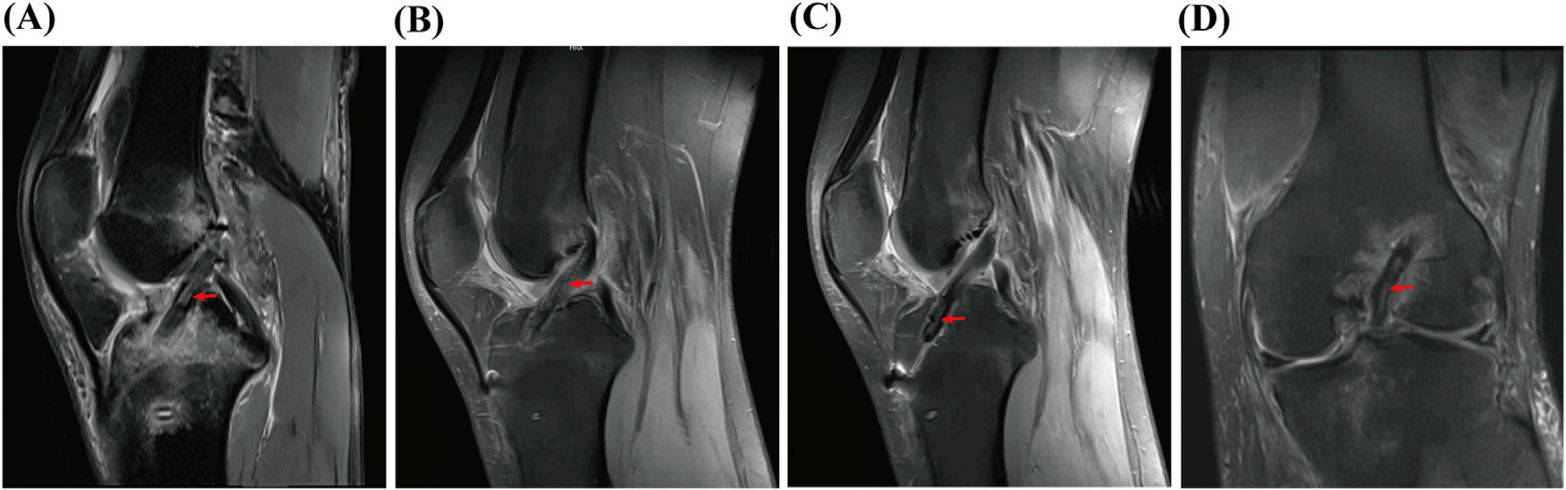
MRI results. (A-C) are sagittal MRI images. (D) is a coronal MRI image. Red arrow: the position of the augmented ligament.
